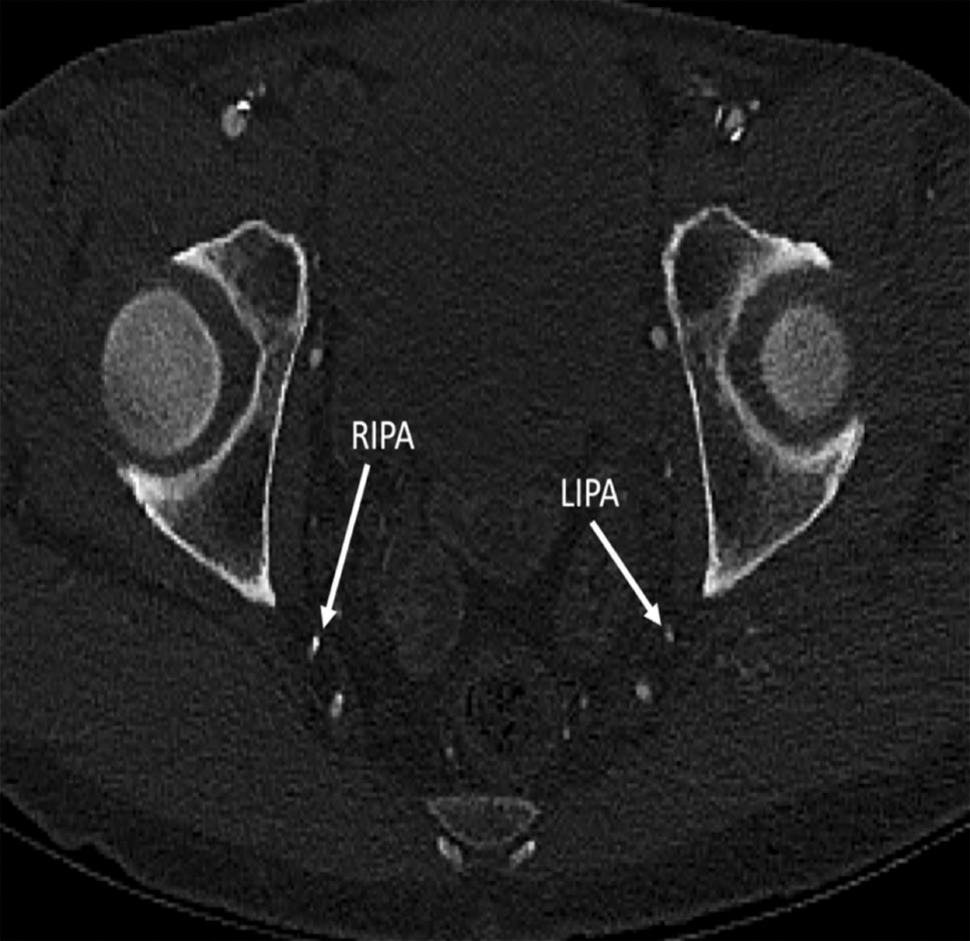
Post-contrast CT images of a 52-year-old male smoker, who scored 10/10 on calcium scoring. Heavy arterial vascular calcification was demonstrated within multiple vessels bilaterally, including the proximal and distal abdominal aorta, common iliac arteries, internal and external iliac arteries, and the internal pudendal arteries. RIPA/LIPA right internal pudendal artery/left internal pudendal artery
